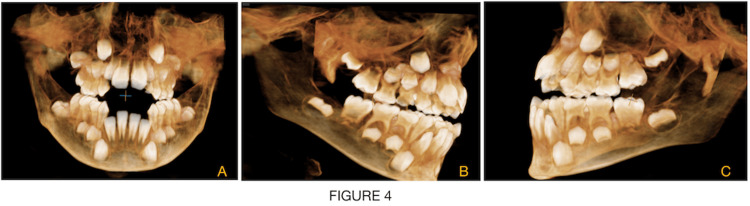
CBCT (cone beam computed tomography) scan confirms the Nolla stages of teeth development. 4A - front view, 4B - right lateral view and 4C - left lateral view
